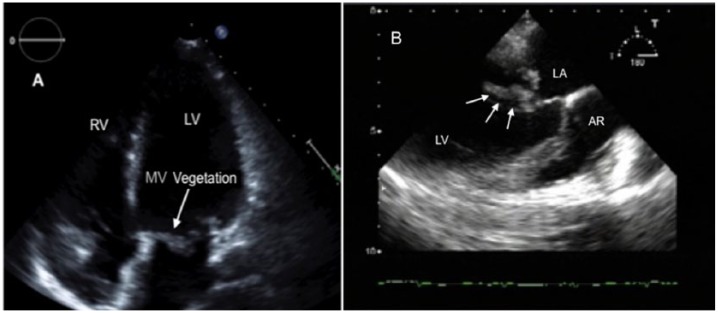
Transthoracic echocardiogram (A) showing a considerable vegetation on the MV. Transesophageal echocardiographic image (B) displays native MV endocarditis. The white arrows show a mobile 23-mm echodense structure on the anterior MV leaflet. MV, mitral valve; RV, right ventricle; LV, left ventricle; LA, left atrium; AR, aortic root.
